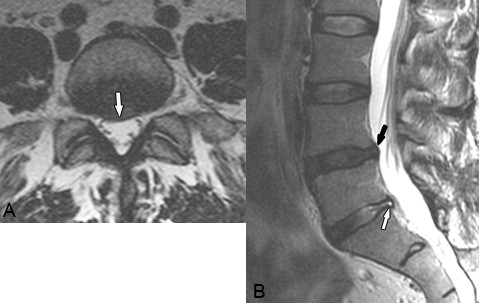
Disc Bulge. A. Axial and B. Sagittal T2-weighted images reveal a focal right-central disc bulge at L4-5 that slightly indents the thecal sac and extends into the right nerve root canal (white arrow on A, black arrow on B). A more focal protrusion and associated annular tear is present at L5-S1 (white arrow on B). While degenerative changes such as disc bulges are extremely prevalent, the only degenerative feature associated with LBP is spinal stenosis. While disc herniations such as protrusions, extrusions, and sequestrations are more likely to result in direct nerve compression and chemical radiculitis resulting in lower extremity symptoms, they occur less frequently in elderly patients. (Images courtesy of Brian A. Howard, Charlotte, NC).
